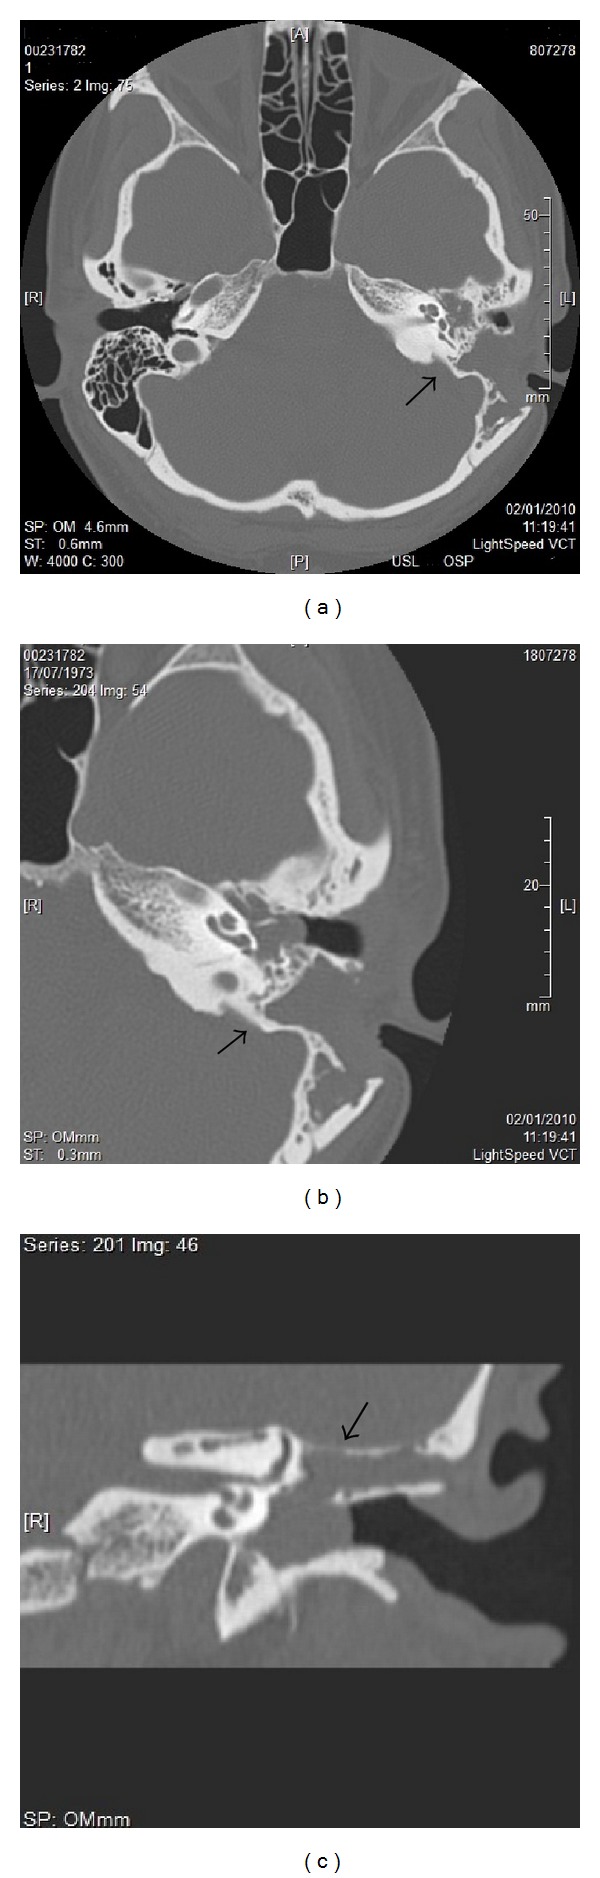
CT imaging (a) with axial (b) and coronal (c) scans demonstrated an irregular, hypodense, and expansile lytic lesion of the mastoid process of the left petrous bone extending to involve both the left medial mastoid and the middle ear, with a subtotal erosion of ossicular chain and a partial bone destruction of the “tegmen tympani” (see arrows). The mass did not appear to affect the semicircular canals, the canal of the facial nerve, and the internal auditory canal.
